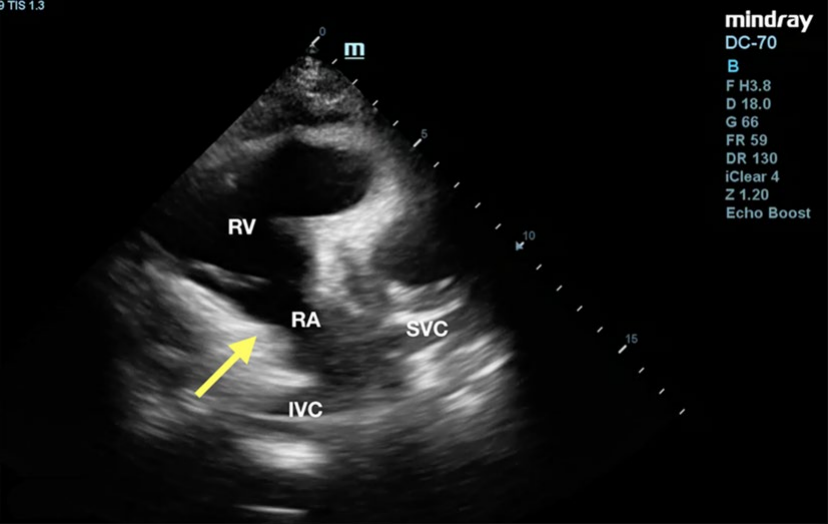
Transthoracic echocardiogram (TTE) of right ventricle inflow view showed a small and compressed right atrium (yellow arrow). Posterior pericardial clot is not well visualized in this view. *IVC* inferior vena cava, *RA* right atrium, *RV* right ventricle, *SVC* superior vena cava
